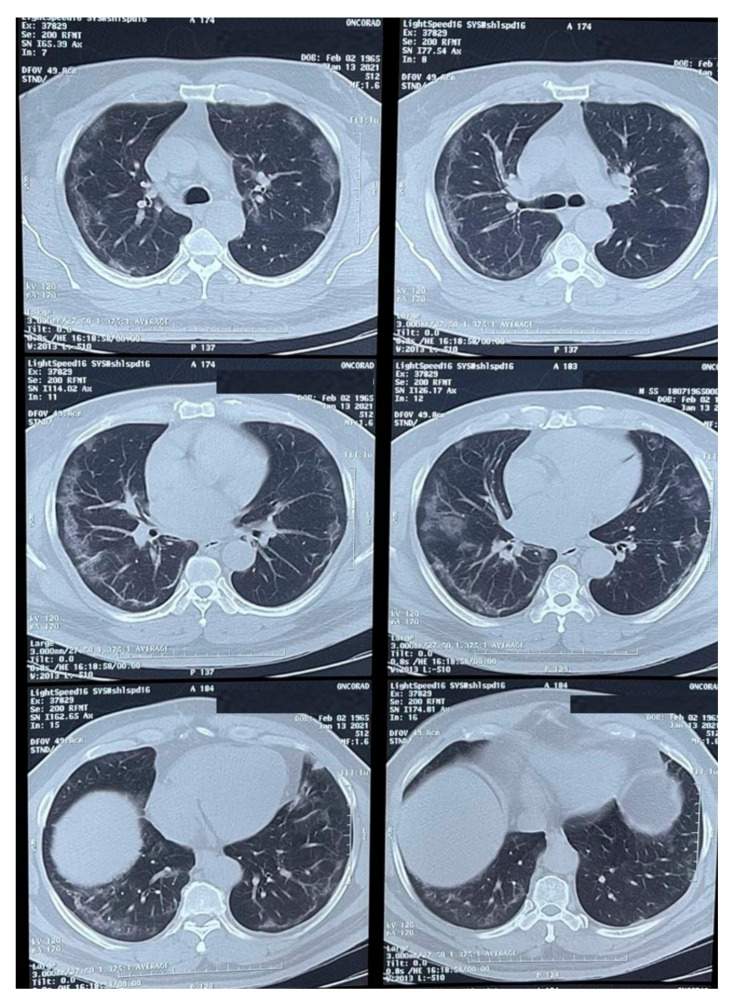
CT-scan of the thorax showing peripheral ground-glass opacities with a partial occupation and consolidations.
